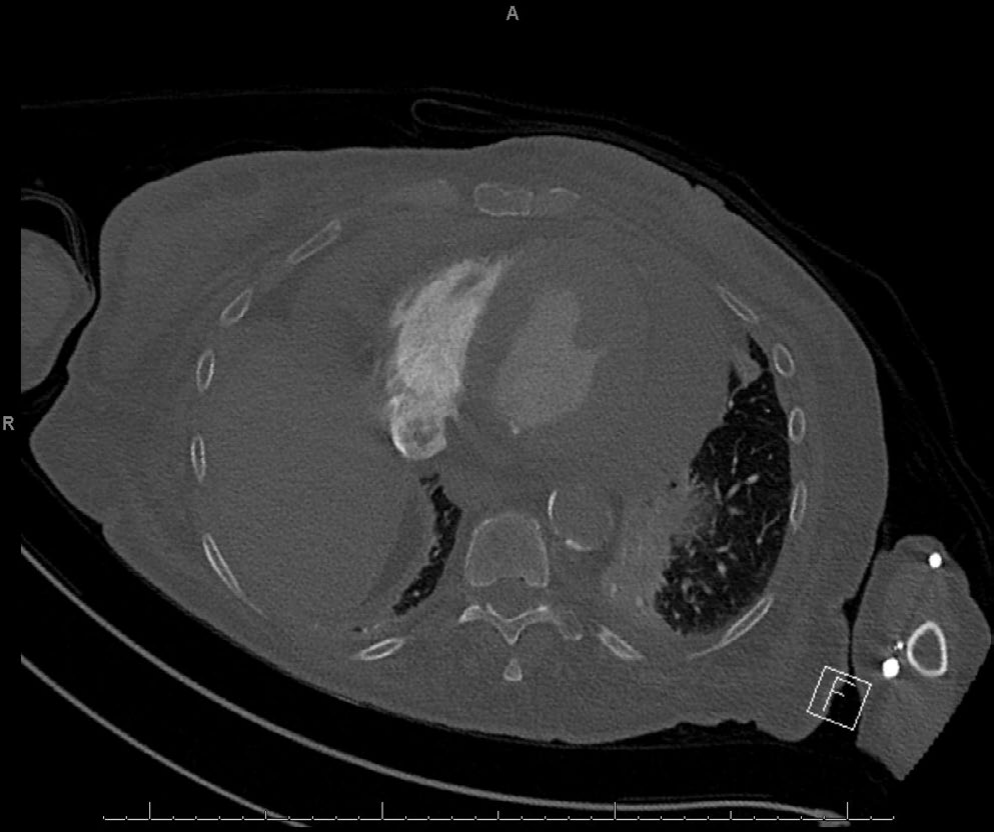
Computerized Tomography (CT) demonstrating large pericardial effusion
